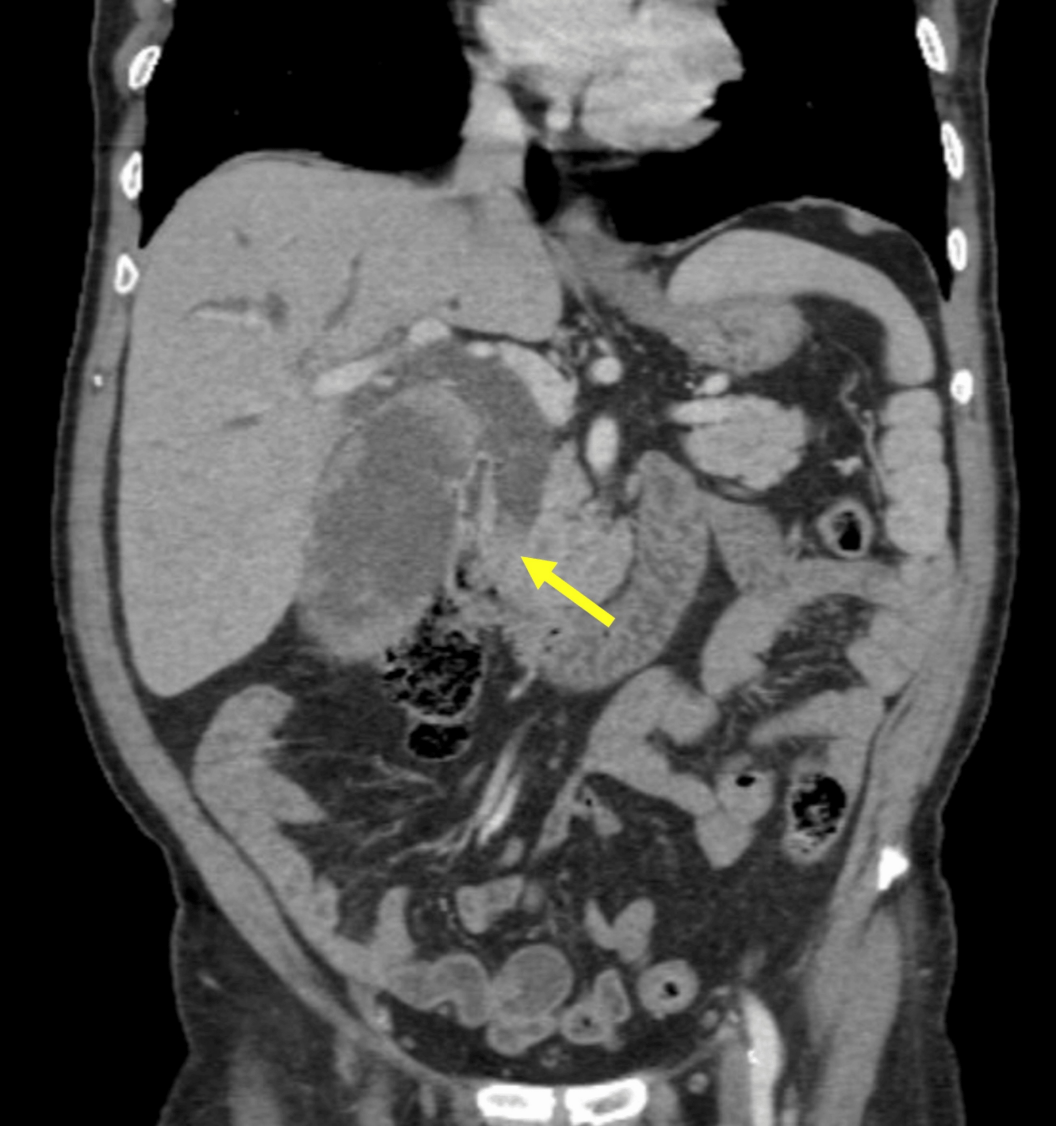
Coronal contrast-enhanced CT of the abdomen demonstrates diffuse dilation of the common bile duct with abnormal enhancement within the distal common bile duct (yellow arrow).
